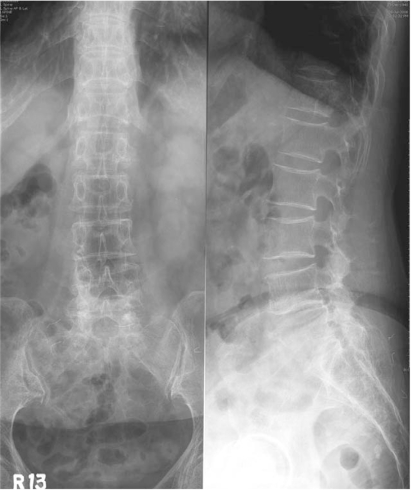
An anteroposterior and lateral radiograph of the lumbar spine shows the multilevel spondylosis with mild osteopenia of L5 body.
